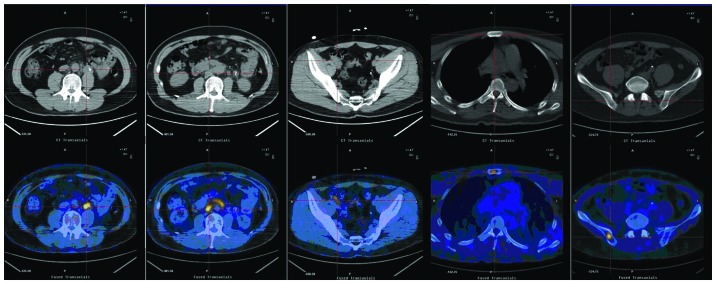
PET-CT scans revealed a nodular soft-tissue density area in the wall of the left-middle ureter at the L3 level, paraaortic and iliac lymphadenopathy and increased FDG uptake in the sternum and right ilium. PET-CT, positron emission tomography-computed tomography; FDG, fludeoxyglucose.
